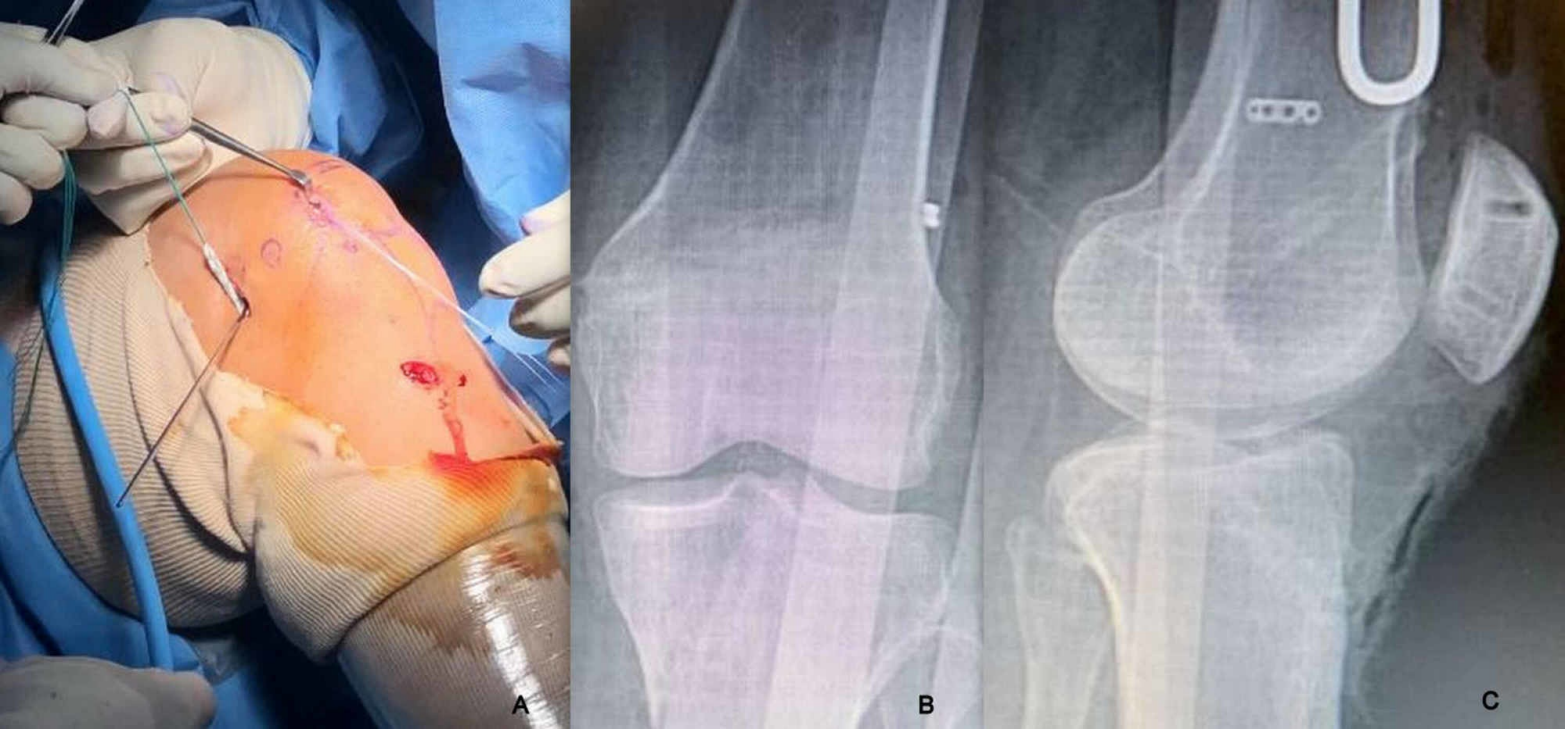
A) Clinical image of gracilis graft being tunneled under layer 2 and secured to femur isometric point; B) Immediate postoperative AP radiograph; C) Immediate lateral radiographs showing proper tunnel positions. AP, anteroposterior
